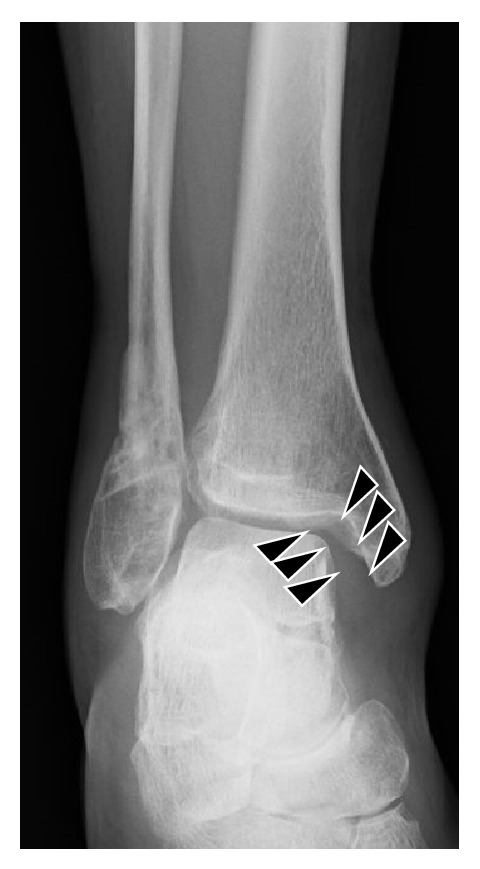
Radiographs at the time of admission to our hospital, after the removal of screws. A shortening of the fibula relative to the medial malleolus, widening of the ankle fork, and a valgus tilt of the talus causing an abnormally widened medial clear space (black triangle).
